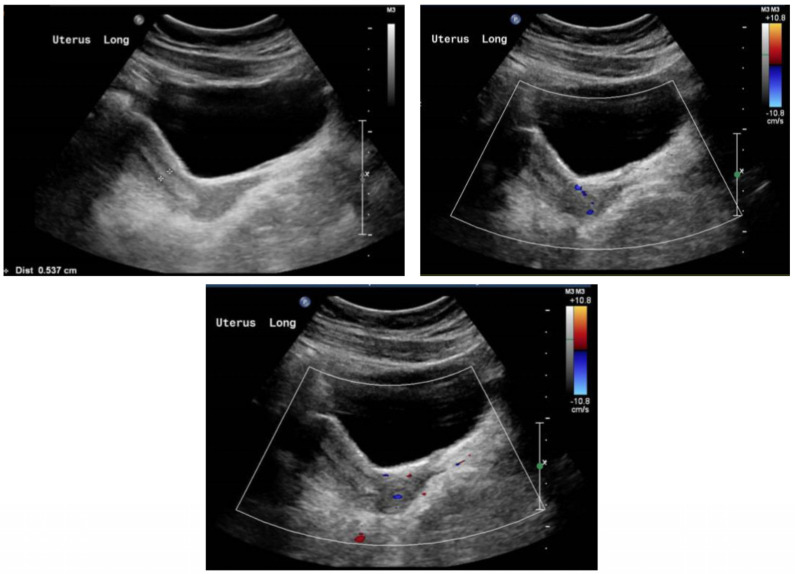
Ultrasound of the uterus following the management plan at 19 years old.
